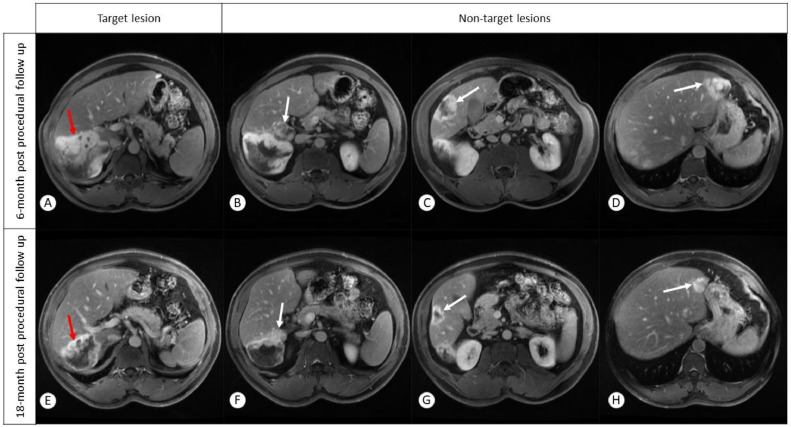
Dynamic MRI of the Case 1 in portal venous phase, showing size reduction of target and nontarget hepatic hemangiomas after direct sclerotherapy. The upper row depicts the 6-month post-DS follow-up and the lower row shows the corresponding changes for each lesion at 18-month post-DS follow-up. The target lesion (red arrows in A and E) shows a significant decrease in size, as well as 3 other nontarget hemangiomas (white arrows).
